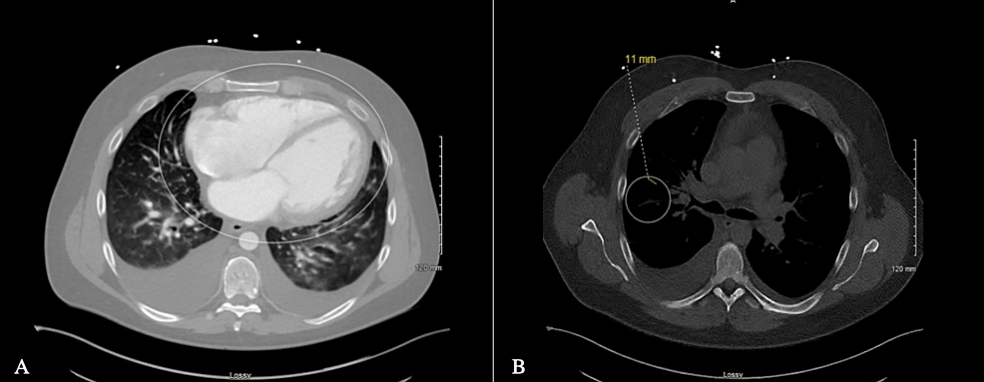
Computed tomography angiogram showing (A) moderate cardiomegaly, (B) 11 mm ground glass opacity in the upper lobe of the right lung
